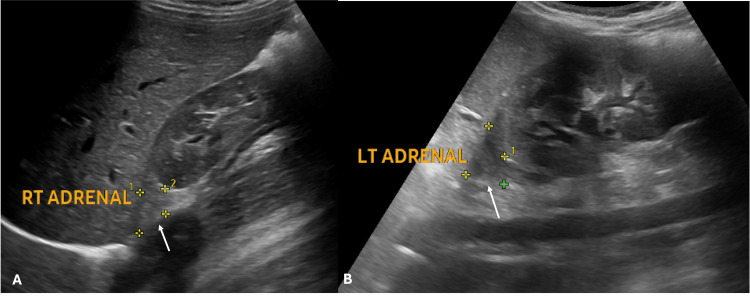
Abdominal ultrasound demonstrating bilateral adrenal hyperplasia (arrow) in a paediatric patient with congenital adrenal hyperplasia, with no detectable testicular lesions (A) Right adrenal gland showing hyperplasia (arrow).
